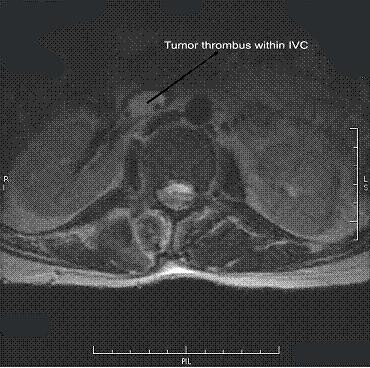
Magnetic resonance imaging scan showing tumor embolus within the inferior vena cava.
